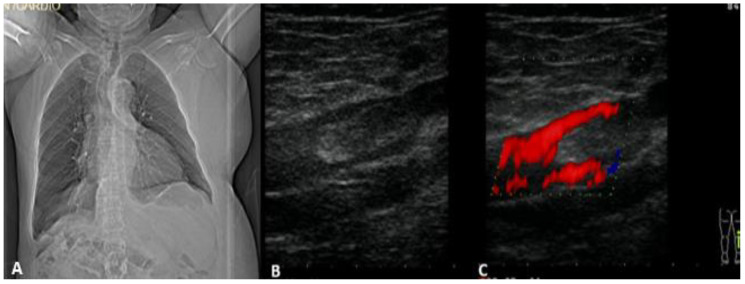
(A) Chest computed-tomography evidencing abdominal situs inversus; (B) Immage of a thrombus in the vein; (C) venous Doppler exam evidencing a thrombus in thevein.
